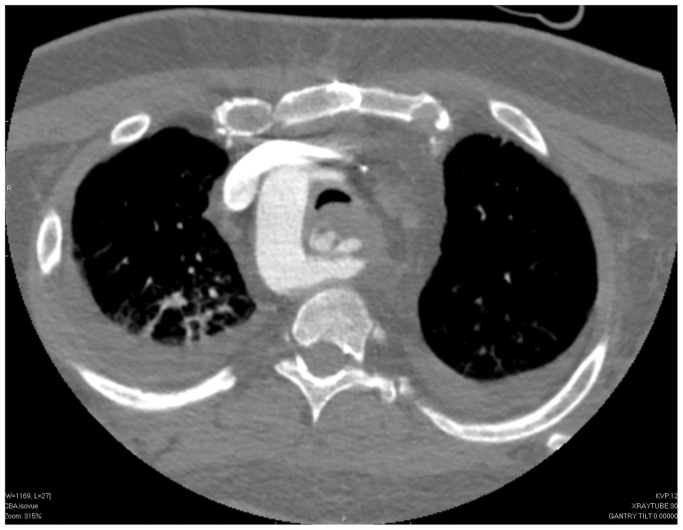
Axial computed tomography angiography (CTA) showing the left subclavian artery-esophageal fistula.
